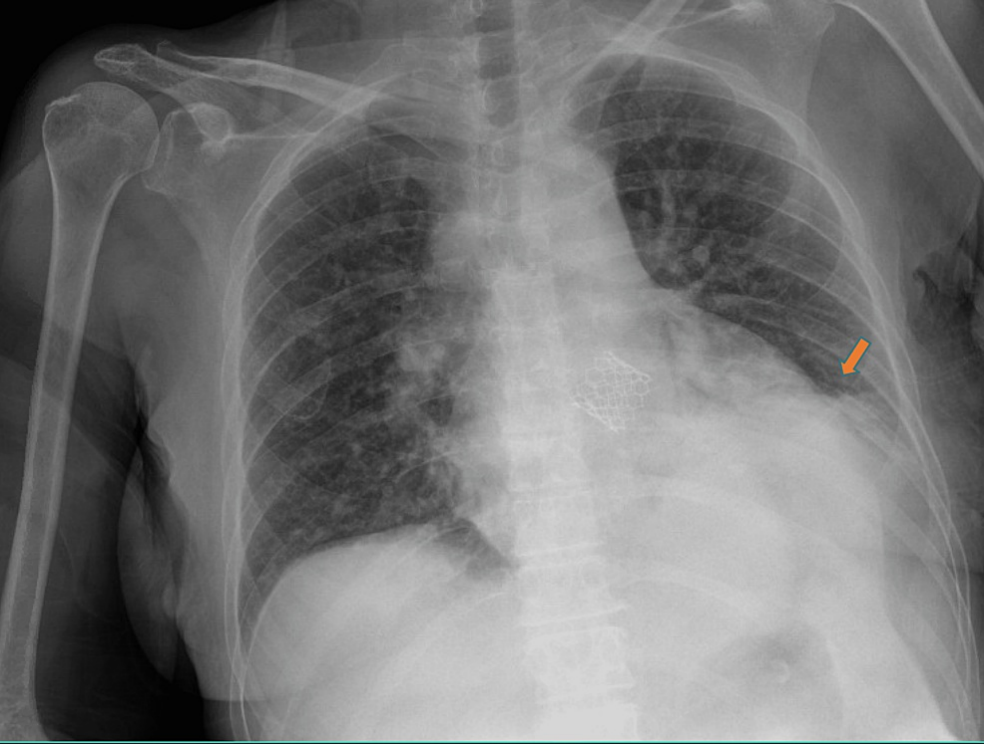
Chest x-ray Anteroposterior view - Left basilar opacity and elevation of the left diaphragm (indicated by the arrow)
